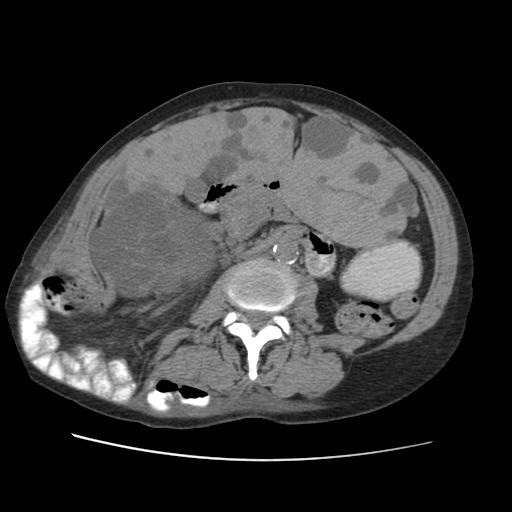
Axial CT scan demonstrating incisional lumbar hernia.
